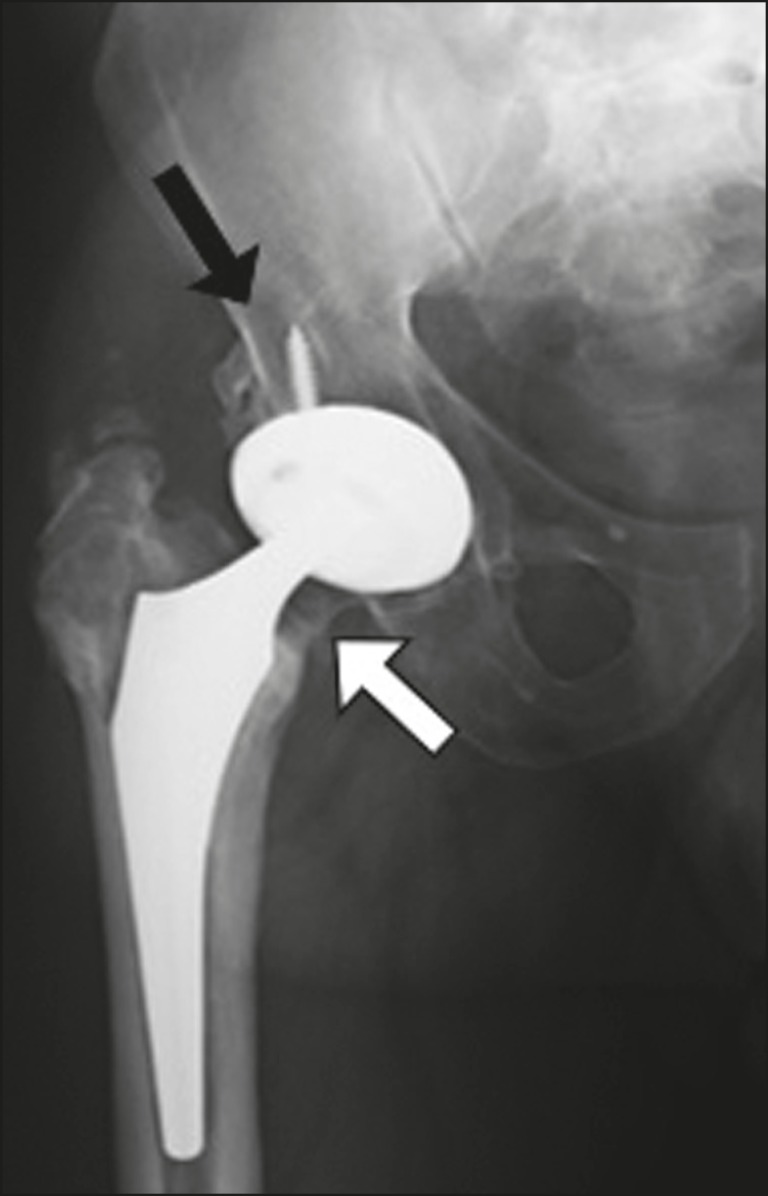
Anteroposterior X-ray of the right hip showing a total bipolar prosthesis and minor resorption (osteolysis—black arrow) of a screw in the acetabular component. Grade IV heterotopic ossification between the femur and the lower border of the acetabulum (white arrow). Cortical thickening without periosteal reaction (stress shielding) is also observed.
